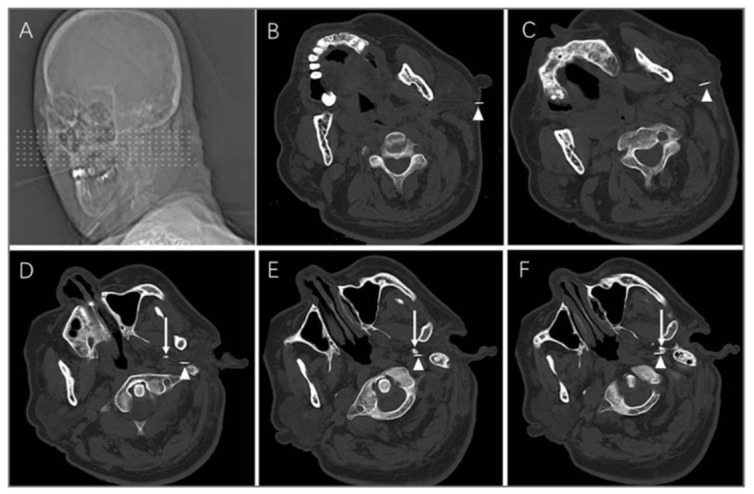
(A) Puncture site for glossopharyngeal nerve access. (B–F) Sequential CT images demonstrating advancement of the needle to the medial margin of the styloid process. White arrows indicate the needle, and white triangles indicate the styloid process. (Reprinted/adapted with permission from Ref. [176] Jia et al. 2020. This figure is published under the Creative Commons Attribution–NonCommercial (CC BY-NC) License, which permits noncommercial use, distribution, and reproduction in any medium, provided the original authors and source are credited).
